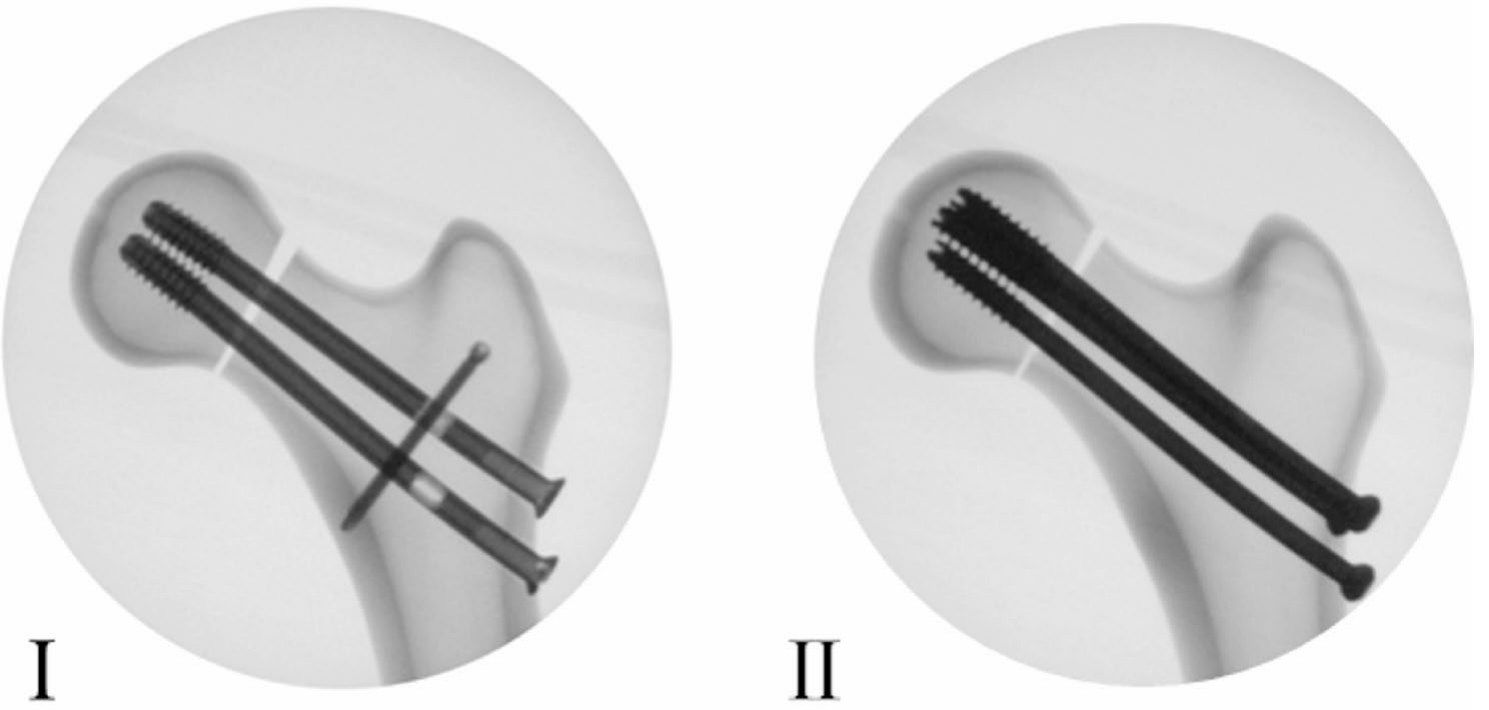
Radiographic example of Sawbones instrumented with (I) the SimpliFix (SF) construct and (II) the inverted triangle construct
